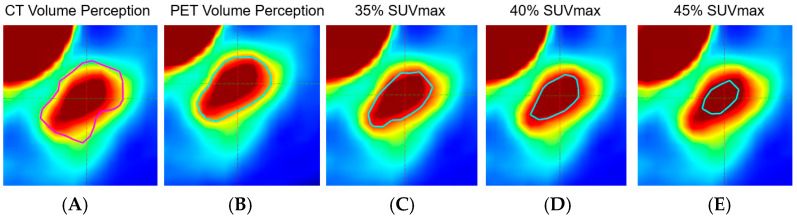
Contour variation based on (A) volume perception on CT; (B) volume perception on PET; (C) 35% of SUV_max_; (D) 40% of SUV_max_; (E) 45% of SUV_max_. The color scale in the PET scans represent levels of metabolic activity: red and yellow indicate high activity, and green and light blue show moderate or low activity.
